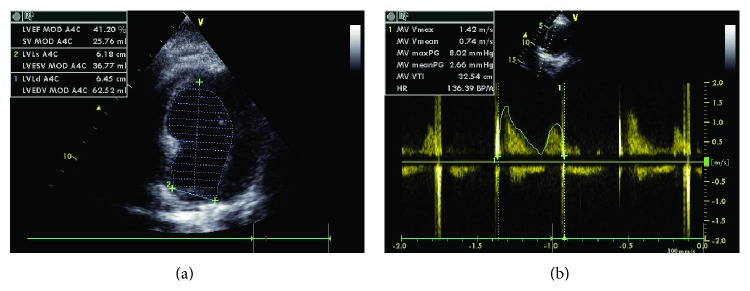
(a) Hospital discharge transthoracic ECHO showed a significant improvement of left ventricular EF, 41%, (b) 1.42 of aortic valve area and Vmax of 2.66.
